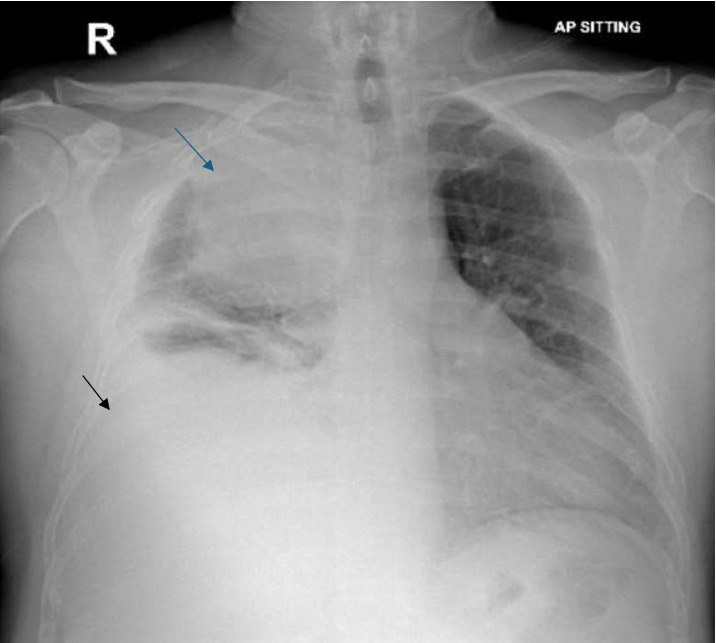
Chest X-ray reveals a homogeneous opacity in the right upper mid-zone, associated with silhouetting of the right heart border (blue arrow), accompanied by moderate right-sided pleural effusion (black arrow).
